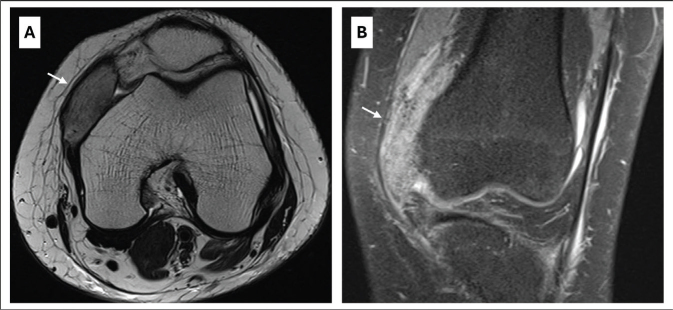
Magnetic resonance imaging of the left knee in a 26-year-old woman without previous relevant medical history, showing extensive heterotopic ossification in the medial femoro-patellar recess with a slight extension to the vastus medius muscle (white arrows). (A) Transversal T2-weighted fast spin echo and (B) Coronal PD-weighted fast spin echo with fat-saturation.
